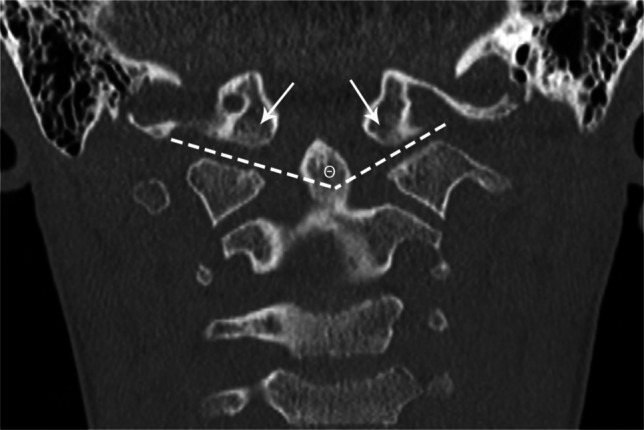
Coronal bone window CT in an 11-year-old female demonstrates abnormally small occipital condyles (*arrows*) compatible with condylar hypoplasia. The atlanto-occipital joint axis angle (*Greek letter theta*), the angle between lines drawn along the atlanto-occipital joints (*dashed lines*), suggests condylar hypoplasia if >127°
